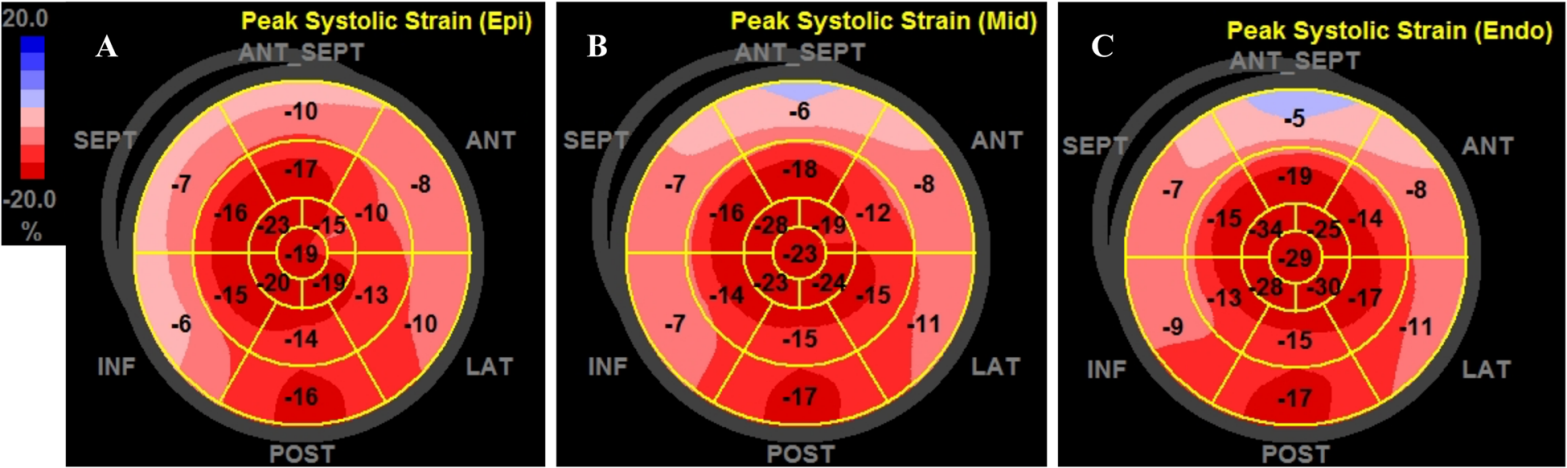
Representative bull’s-eye map of a patient with hypertrophic cardiomyopathy. A multilayer bull’s-eye map, showing the epicardial (a), middle (b), and endocardial myocardial layers (c), was automatically generated after the layer-specific strain was measured in 3 apical views (apical 4-, 2-, and 3-chamber views). An 18-segment model was used in the segmental analysis
